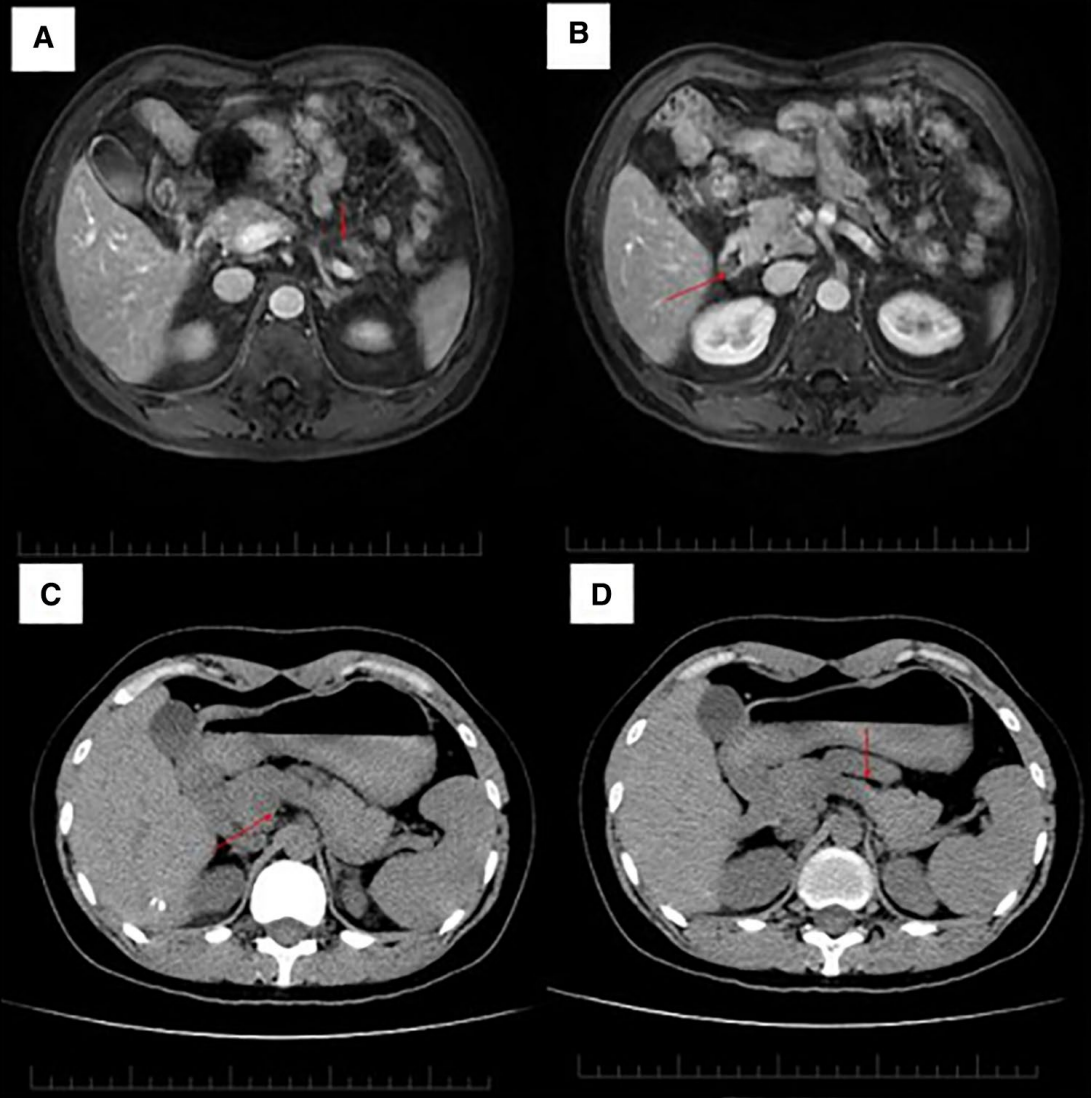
Imaging of pancreas in the proband and his daughter. Magnetic resonance imaging of the proband showing only the head of the pancreas is visible (A, B). Computed tomography scan of his daughter with pancreatic body hypoplasia (C, D). Abnormalities are indicated by red arrows
